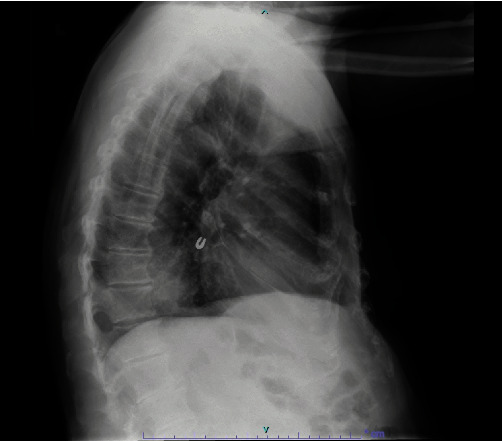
Lateral chest X-ray demonstrating radiopaque foreign body.
